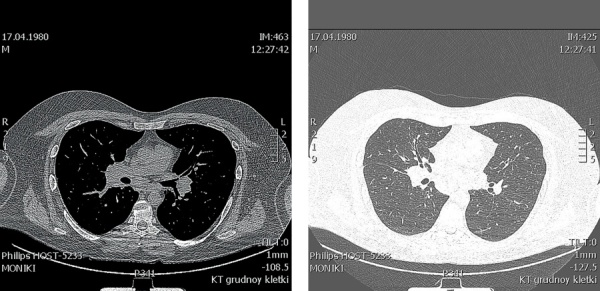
Рисунок 4, 5. КТ органов грудной клетки от 2018г.(Увеличение лимфоузлов средостения (паратрахеальных и бифуркационных)). Figure 4, 5. CT scan of the chest organs from 2018(Enlarged mediastinal lymph nodes (paratracheal and bifurcation))
